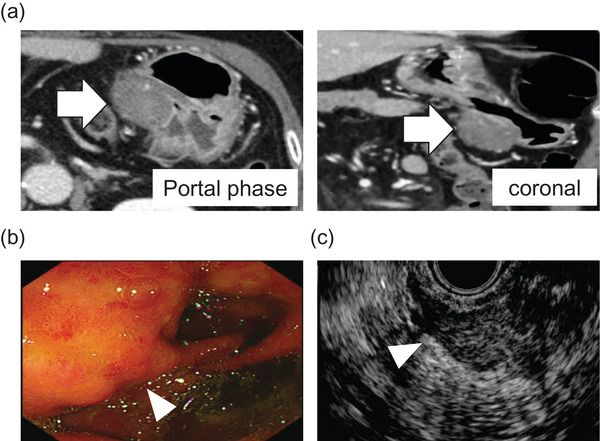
(a) CT scan at 2 years post‐preoperative for pancreatic cancer. Arrows (⇒) indicate that the mass appeared at the same site as the EUS‐FNB puncture, one to the primary pancreatic cancer, NTS was suspected. (b, c) Arrowheads (▷) indicate the mass in (b) esophagogastroduodenoscopy and (c) EUS. (b) Endoscopic images of the mass. (c) EUS‐FNB for the mass. CT, computed tomography; EUS‐FNB, Endoscopic ultrasound‐guided fine‐needle biopsy; NTS, Needle tract seeding.
